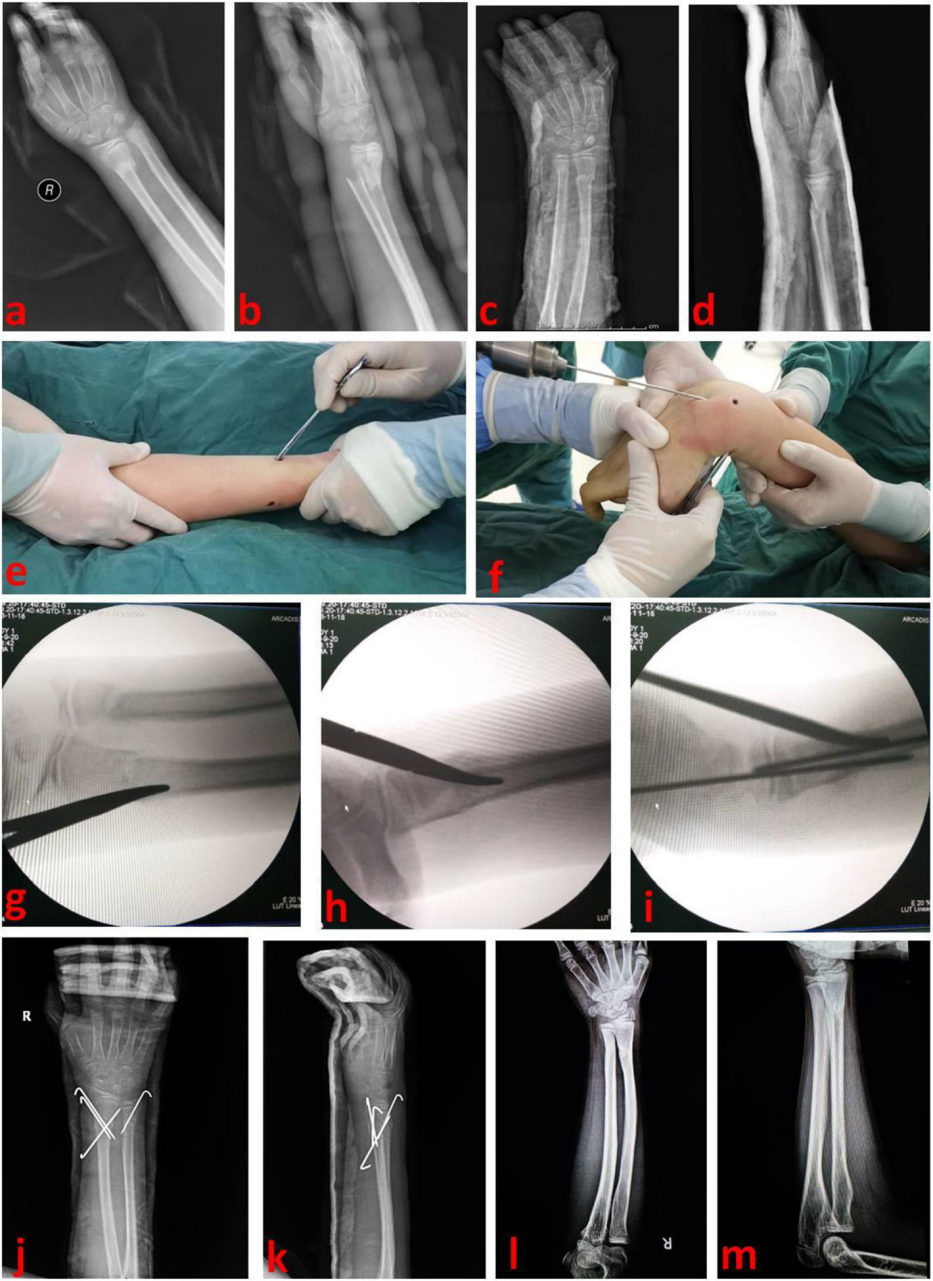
An 11-year-old child suffered from distal radius-ulna fracture and was reduced by PRMF technique. a and b: Preoperative X-ray films showed distal radius-ulna fracture of right forearm; c and d: There was still obvious fracture displacement after manual reduction and plaster fixation; e: Intra-operative prying and manual reduction; f: During the operation, reduction was maintained and Kirschner wire fixation was performed; g, h and i: X-ray films showed that, PRMF technique was used for reduction and maintaining reduction; j and k: X-ray films after the operation showed that this child achieved good reduction and percutaneous Kirschner wire fixation; l and m: The distal radius-ulna fracture healed well and X-ray films were taken after Kirschner wires were removed. PRMF stands for prying reduction with mosquito forceps
